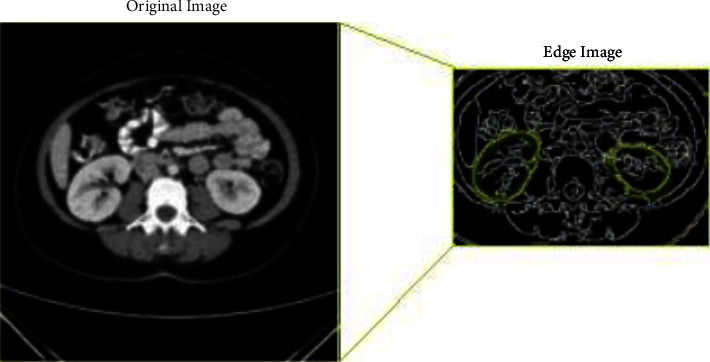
Original input CT images and edge detector output images.
